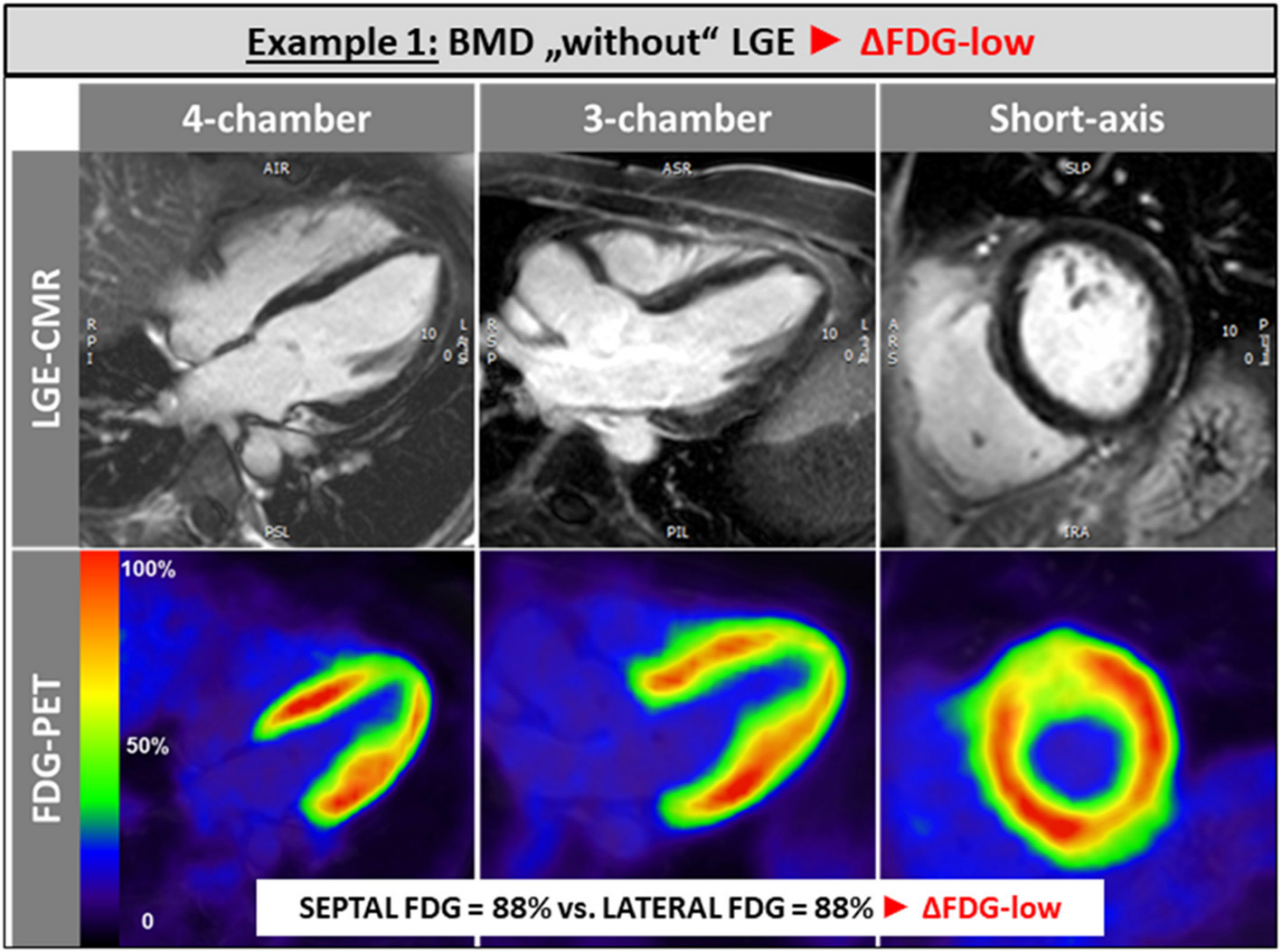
The single patient without any LGE and preserved LV and RV systolic function showed a balanced FDG uptake when septal segments were compared to lateral ones.
